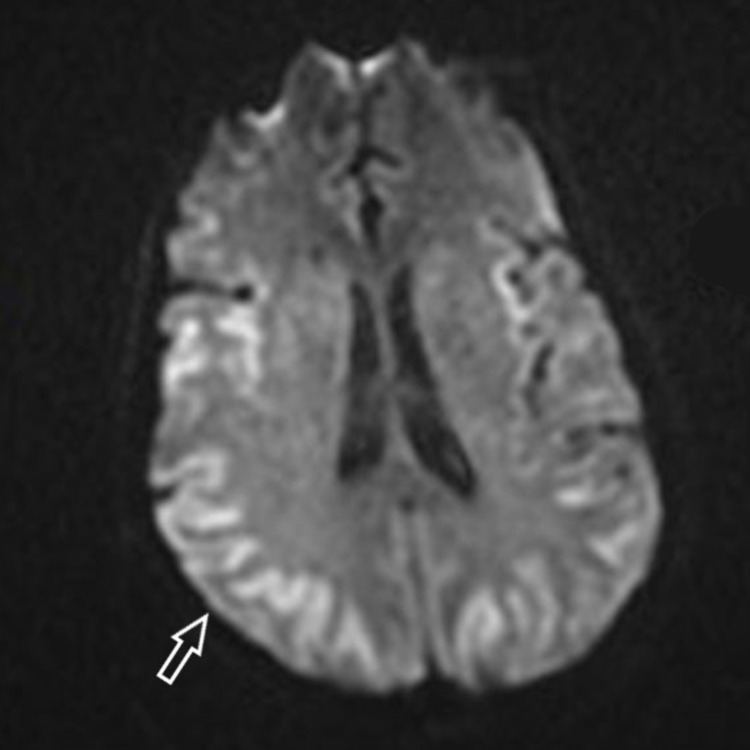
Magnetic Resonance Imaging of the brain showing worsening diffusion weighted abnormalities Arrow over right parietal lobe showing areas of hyperintensity suspicious for hypoxic injury
